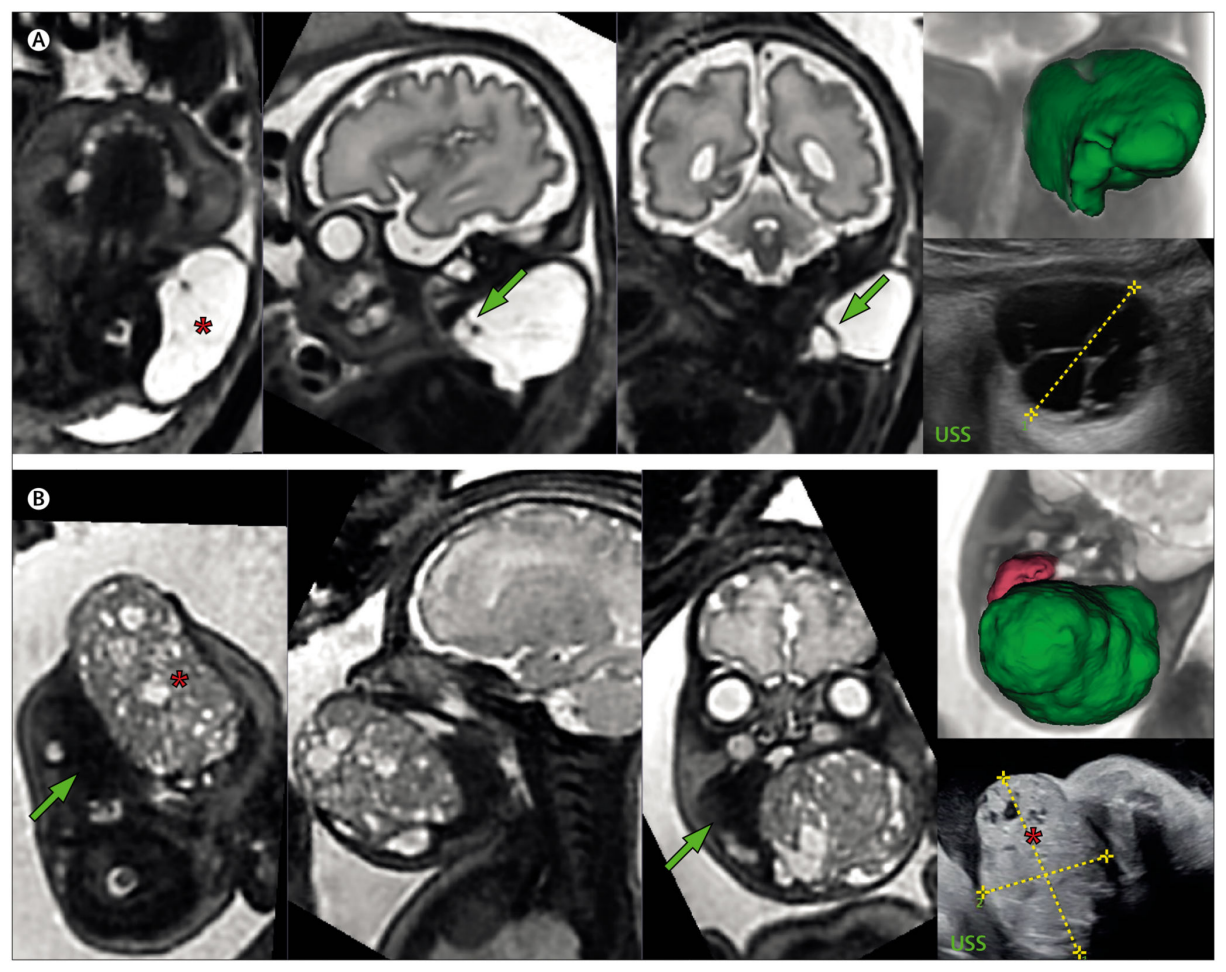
T2-weighted MRIs of two fetuses with neck masses (A) Fetus at 31 weeks and 6 days’ gestation with a lymphatic malformation (marked by an asterisk) of the posterolateral left neck, with arrows pointing to internal septations. The 3D model (top right) shows the lesion (green) lying separate to the fetal airway, major vessels, and CNS structures. The corresponding fetal ultrasound is shown bottom right. (B) Fetus at 33 weeks and 4 days’ gestation with an oropharyngeal teratoma (marked by an asterisk) in relation to the tongue (green arrow). The sagittal and coronal views in the two central images show the relationship to the fetal airway. The corresponding fetal ultrasound is shown bottom right. The 3D model (top right) shows the 85 cm^3^ tumour mass (green) and tongue (pink). 3D=three-dimensional. USS=ultrasound scan.
